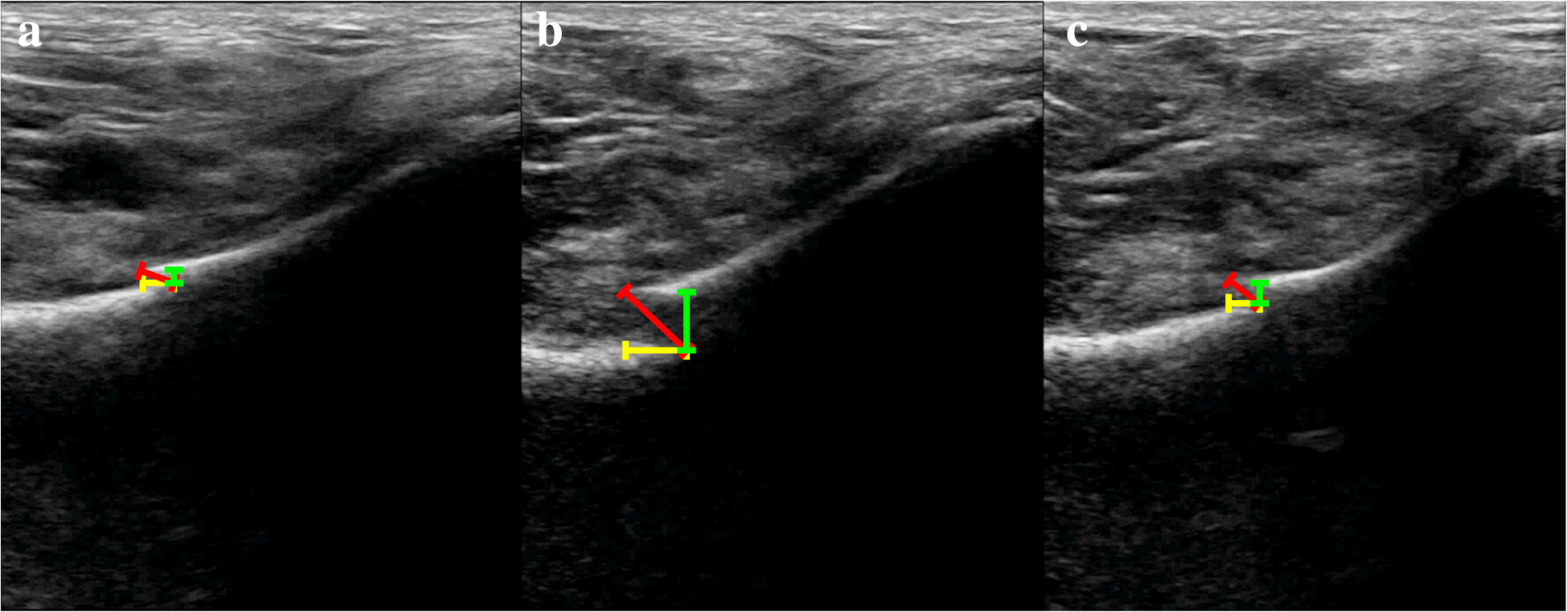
Method of measuring displacement in three directions, performed measures the displacement under the ultrasonic probe while the orthopedist performs the pelvic compression and separation tests. a Displacement at rest. b Displacement during the compression test. c Displacement during the separation test. The yellow line shows the L-R distance, the green line shows the A-P distance and the red line shows the oblique distance
